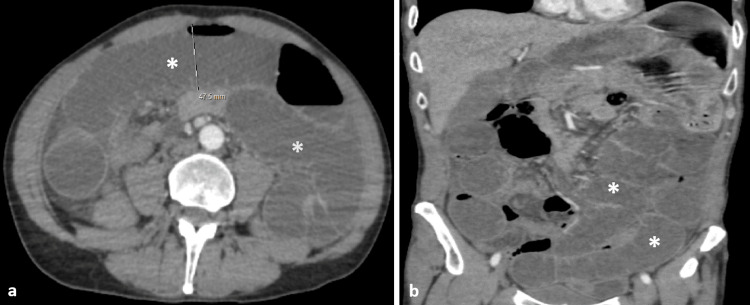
Abdominal CT scan showing markedly distended bowel loops (*) with a coffee-bean appearance, without visible Meckel’s diverticulum. (a) Transverse view and (b) coronal view.
